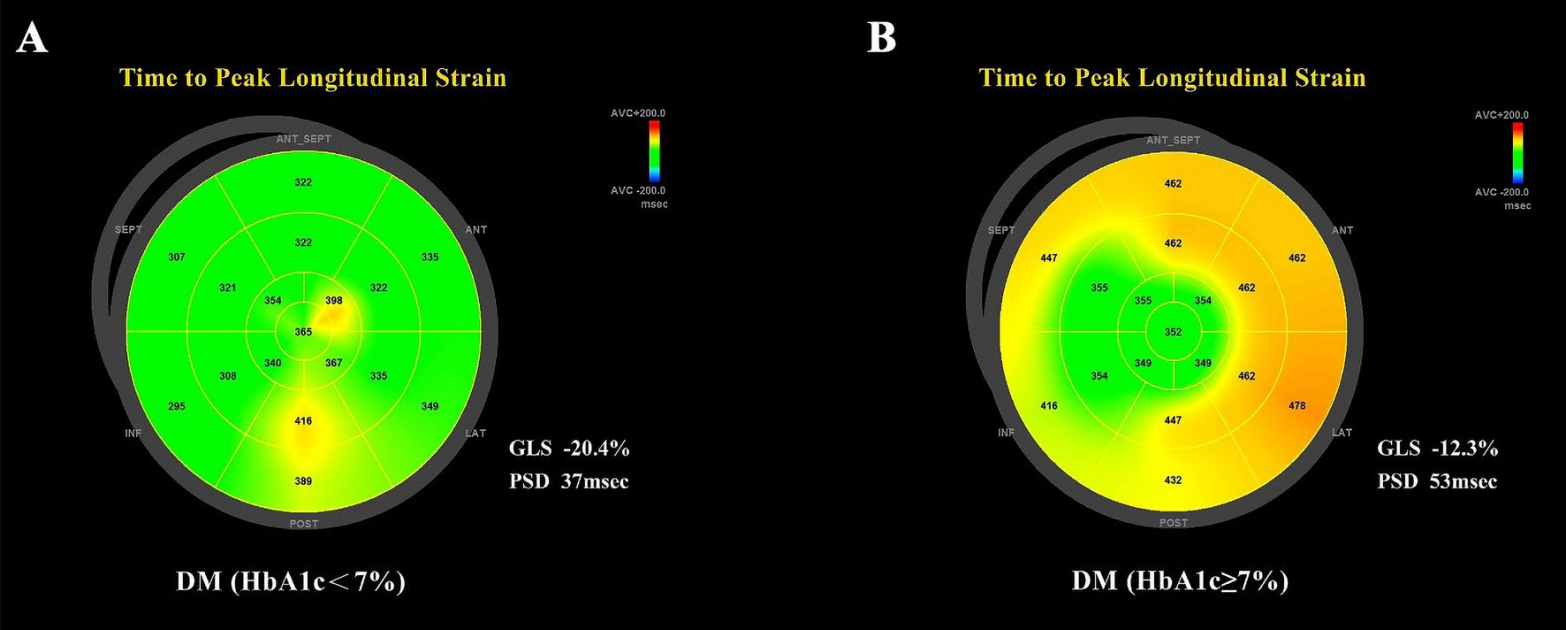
Bullseye plots of time to peak longitudinal strain are shown in two T2DM patients using EchoPAC workstation 2DSTE analysis. The endocardium of the LV was tracked point by point in the apical four-chamber, apical three-chamber and apical two-chamber views. After 17 segments of the LV were successfully tracked, GLS and LV PSD were automatically abtained. A patient with controlled blood glucose (HbA1c < 7%) displayed an LV GLS of 20.4% (absolute value) and LV PSD of 37 ms (A). More pronounced LV GLS of 12.3% (absolute value) and LV PSD of 72 ms were observed in a DM patient with uncontrolled blood glucose (HbA1c ≥ 7%) (B). *AVC* aortic valve closure, *ANT* anterior, *SEPT* septal, *LAT* lateral, *POST* posterior, *INF* inferior.
